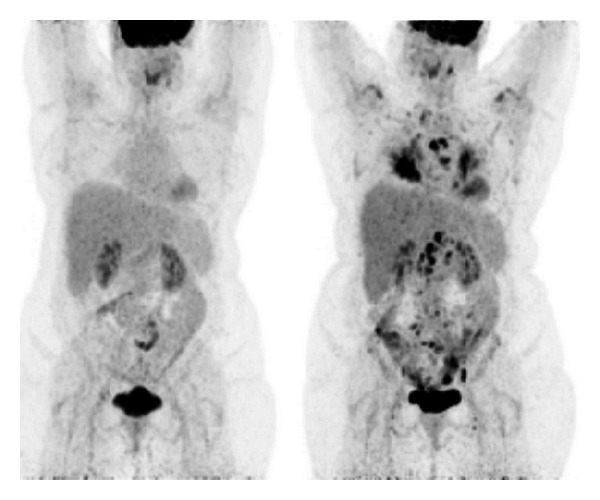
^18^F-FDG-PET scan monitoring sarcoidosis activity level. In the left frame is shown the ^18^F-FDG-PET scan during treatment with infliximab and in the right frame the ^18^F-FDG-PET scan after discontinuation of infliximab revealing reactivation of sarcoidosis as black spots in lungs and lymph nodes.
